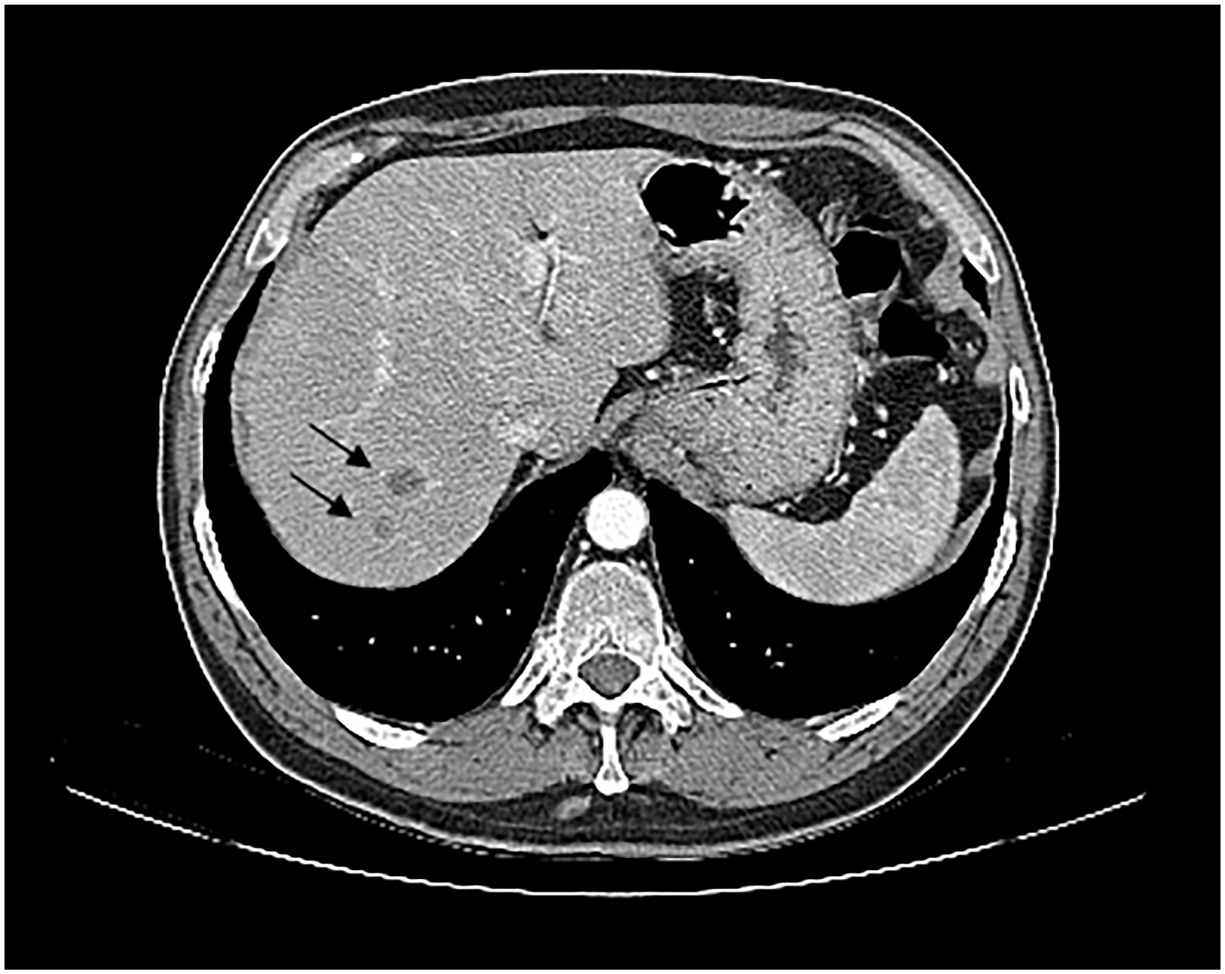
Axial contrast-enhanced CT scan upon progression. Image shows hepatic progression with metastatic lesions not accessible to biopsy (arrow).
